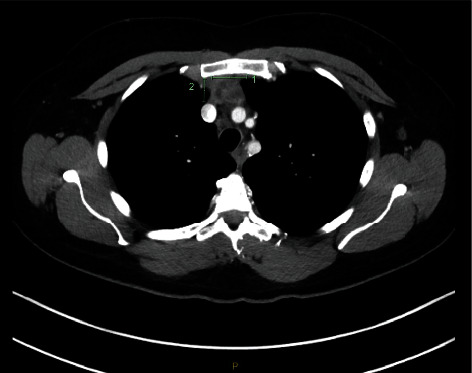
CT scan postchemotherapy treatment showing a reduction in the size of the mediastinal mass.
